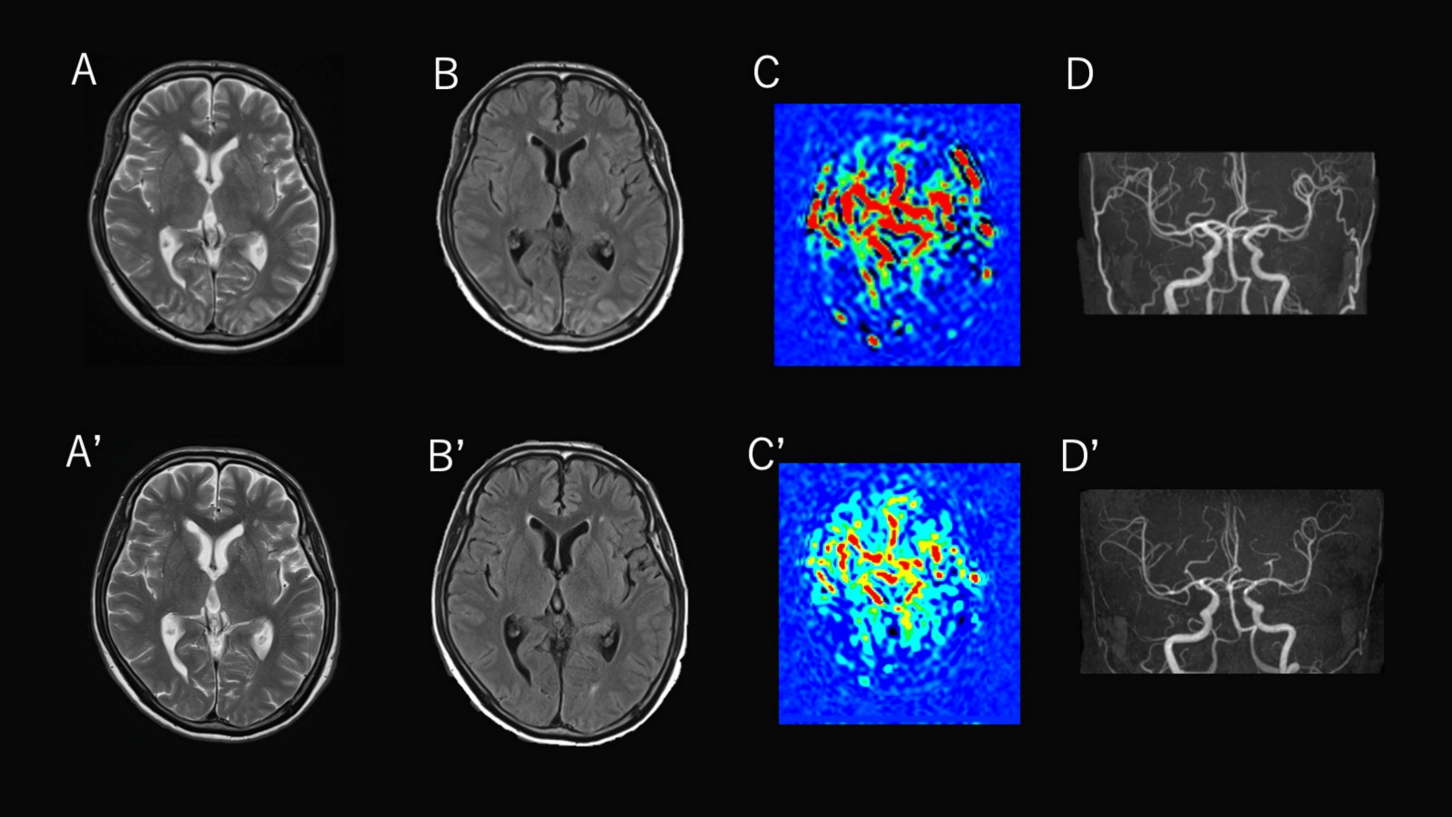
Comparison of brain MRI findings between day 1 and day 10 of hospitalization The upper row shows images obtained on day 1 of hospitalization (A–D), and the lower row shows images obtained on day 10 of hospitalization (A’–D’). T2-weighted (A, A’) and FLAIR (B, B’) images demonstrate that the occipital hyperintensity observed on day 1 of hospitalization resolved by day 10 of hospitalization. ASL (C, C’) shows that the hyperperfusion seen on day 1 of hospitalization disappeared on day 10 of hospitalization. MRA (D,D’) shows that the increased arterial visualization/prominence noted on day 1 of hospitalization also resolved by day 10 of hospitalization. Note: The left side of each image corresponds to the patient’s right side.
